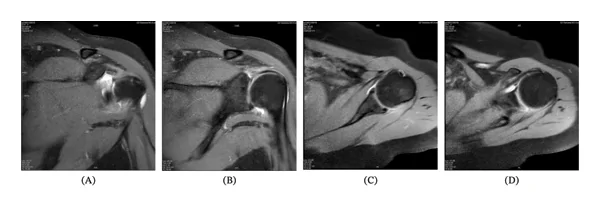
MRI diagnosis of adhesive capsulitis (frozen shoulder) (A) Adhesive capsulitis in the inflammatory phase; LHBT tenosynovitis; mild SASD bursitis. No definite full‐thickness cuff tear. (B) Supraspinatus tendinopathy with a probable small articular‐sided partial‐thickness tear (Ellman Grade I, < 25%–30% thickness). No full‐thickness tear. (C) Supporting adhesive capsulitis; LHBT tenosynovitis; mild subscapularis tendinopathy without definite tear. (D) Rotator interval and coracohumeral ligament involvement—further evidence for adhesive capsulitis; LHBT tenosynovitis. No definite labral tear on this slice.
